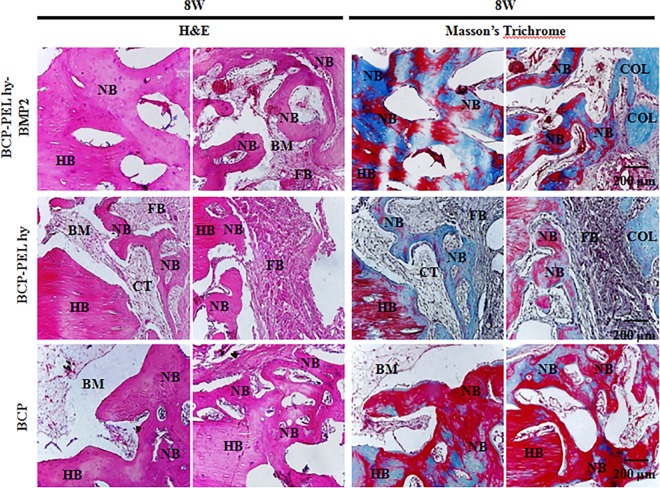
Cellular activity at scaffold-host bone interphase at 8 W time period. Here HB, NB, BM, CT, FB and COL stands for host bone, new bone, bone marrow, cartilage template and collagen.
